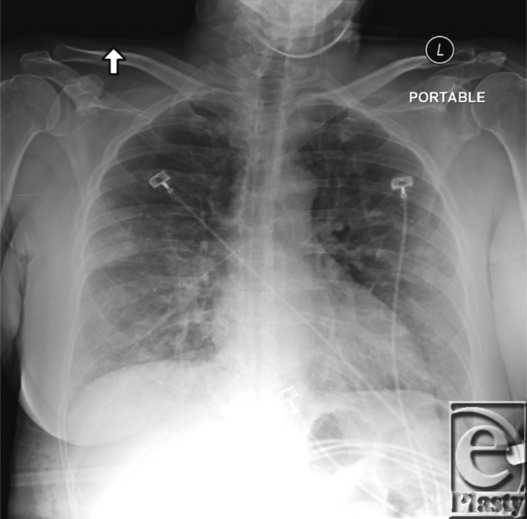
Chest radiograph with bilateral mid and lower lung patchy airspace opacities.
